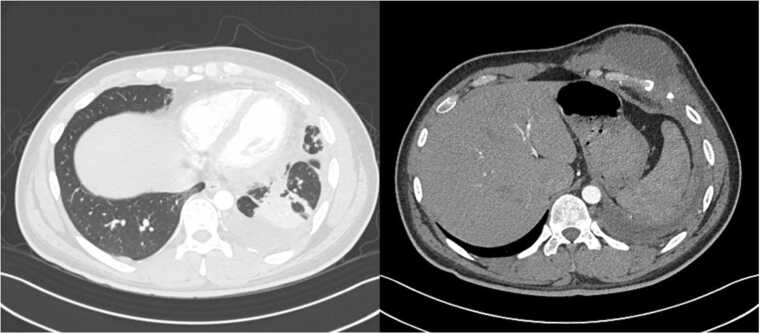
CT Chest/Abdomen/Pelvis demonstrating consolidation and atelectasis in the left lower lobe suspicious for pneumonia with an adjacent loculated pleural effusion concerning for empyema. Soft tissue stranding present through chest wall with effacement of left anterior sixth rib with additional organized fluid collection in lower chest consistent with empyema necessitans.
